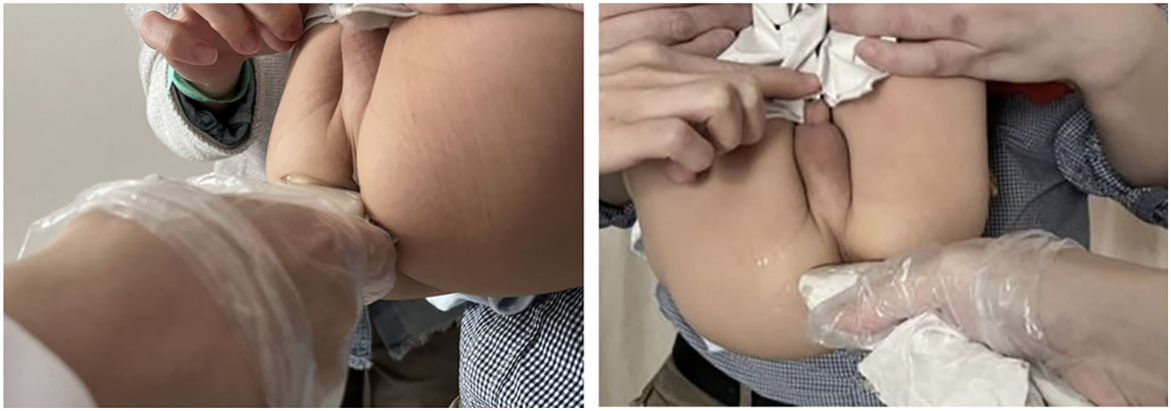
Transcutaneous perianal ultrasound examination
